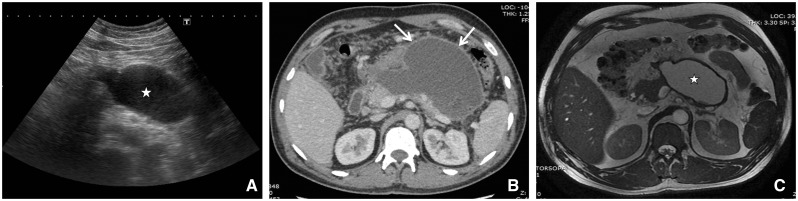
Diagnostic images from a 36-year-old with walled-off necrosis after acute necrotizing pancreatitis. (A) Ultrasound showing collection with well-defined wall and coarse internal echoes (asterix). (B) Axial contrast-enhanced CT showing heterogeneous collection with well-defined wall (arrows). (C) MR axial fast imaging employing steady-state acquisition image (FIESTA) showing WON (asterisk) with thick wall and necrotic debris.
